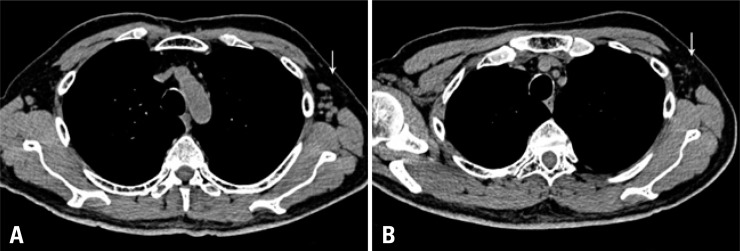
Computed tomography before (4A) and 9 weeks after (4B) I-131 therapy. Note the marked reduction of lymph nodes in the left axilla
